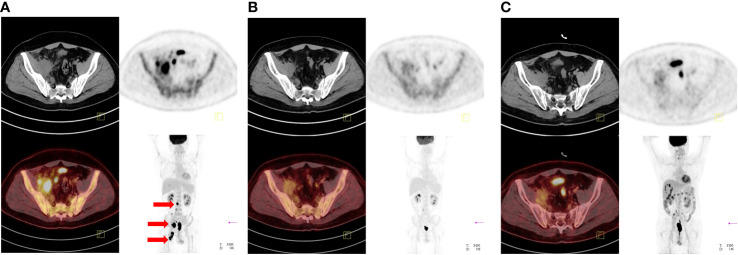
PET-CT evaluation before and after ASCT and CAR T-cell infusion. (A): PET-CT examination demonstrated PD before ASCT and CAR T-cell infusion. (B): PET-CT examination demonstrated CMR in the +1 month after ASCT and CAR T-cell therapy. (C): PET-CT examination demonstrated sustained CMR in the +3 month after ASCT and CAR T-cell therapy. The thick arrows represent the positive lesions; thin arrows represent the original comparable lesion locations.
